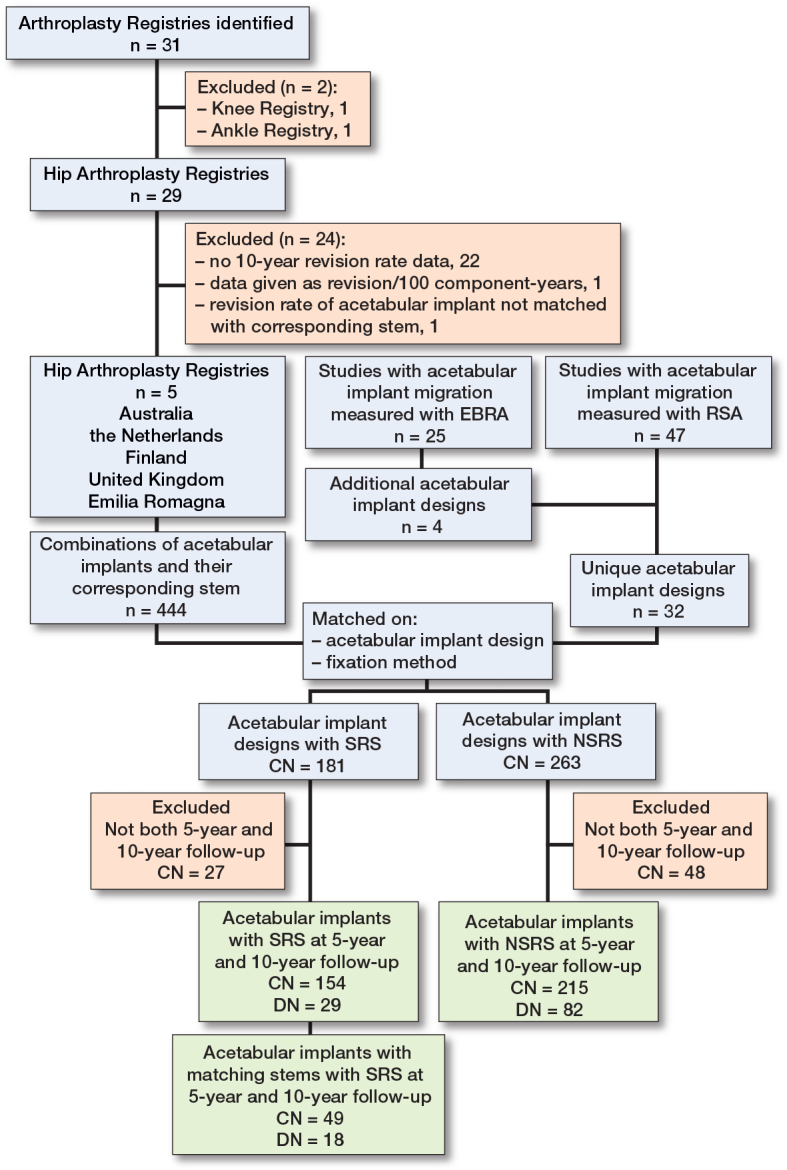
Number of registries and number of acetabular implant designs and combinations included in the study. CN = number of acetabular implant and stem combinations; DN = number of acetabular implant designs; SRS = sensitive radiographic surveillance; NSRS = no sensitive radiographic surveillance.
